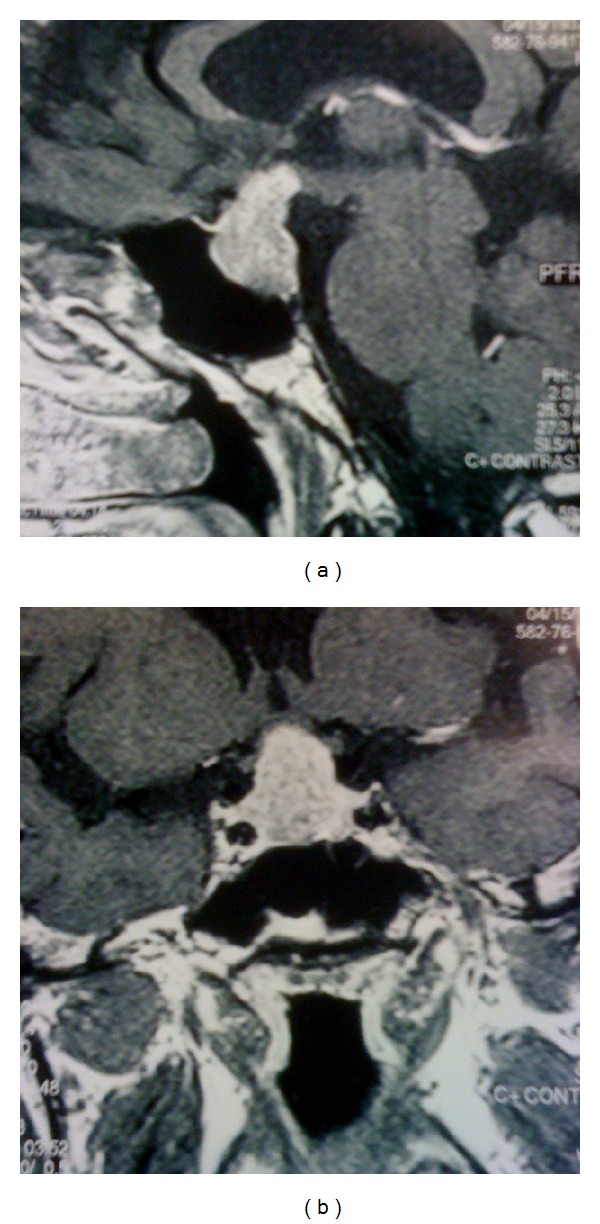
MRI T1-weighted scans on admission: (a) sagittal section, (b) coronal section.
